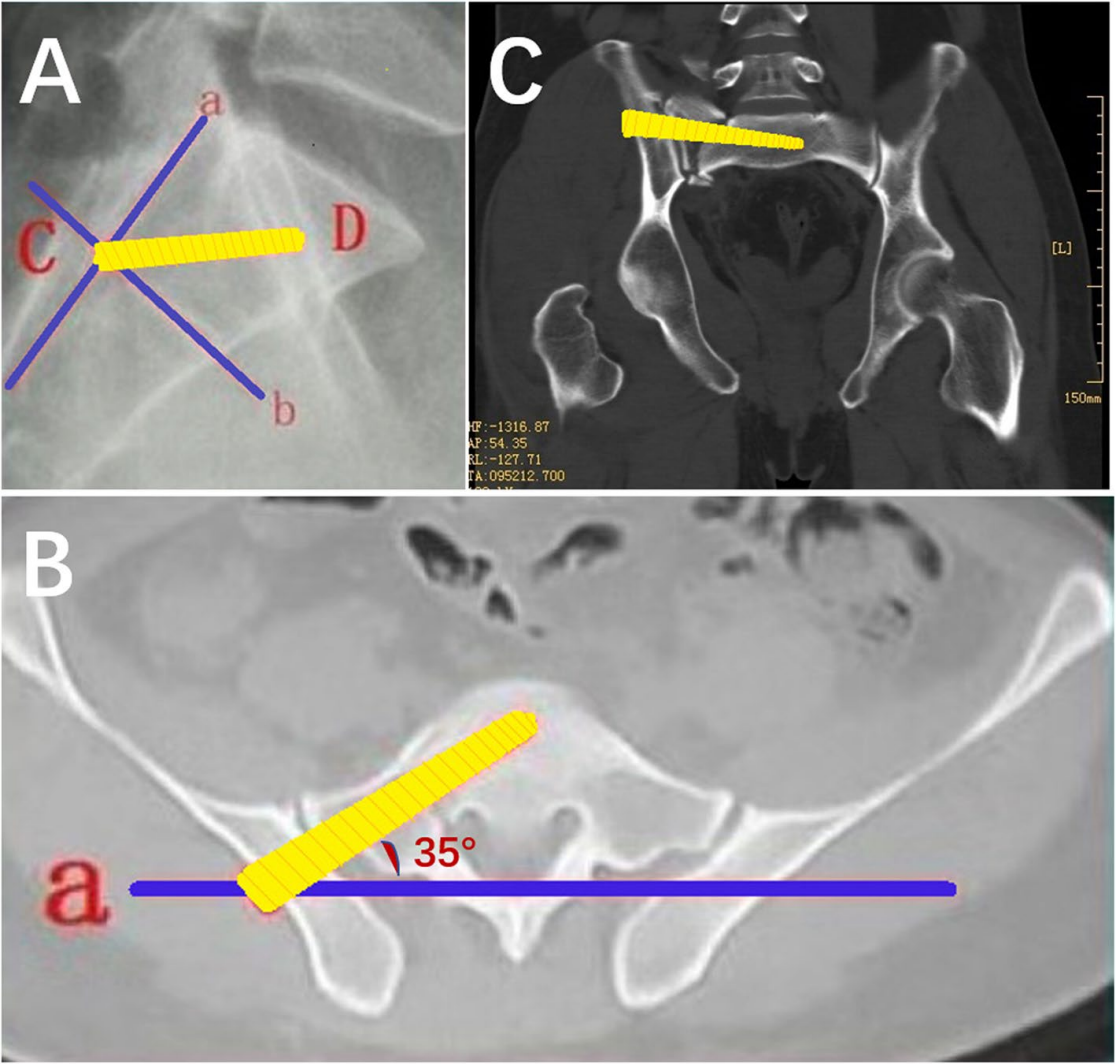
51-year-old male with Tile type B2 pelvic fracture. A: Lateral radiograph shows the nail placement point at the posterior edge of the spinal canal; B: transverse CT shows that the nail placement point was displaced from the anterior to the posterior edge of the spinal canal and was tilted forward by about 35°. C: Coronal CT shows that the screw was oriented perpendicular to the sacral fracture line and located anteriorly about 0.5 cm from the anterior edge of the S1 vertebral body
